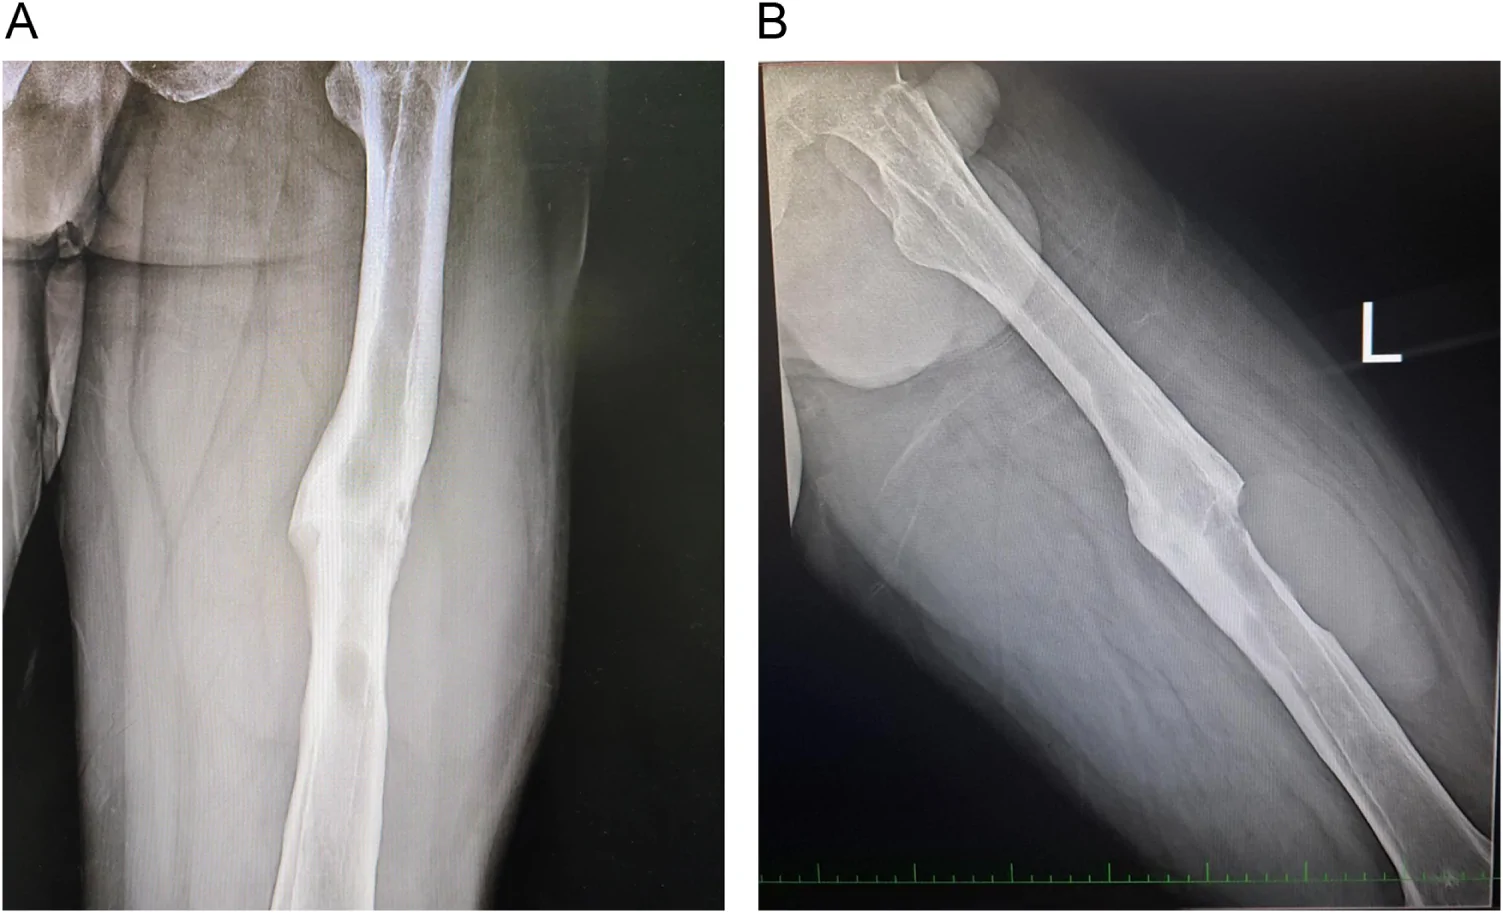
(A and B) Plain radiography of the left femur; anteroposterior view (Fig. 1A) and lateral view (Fig. 1B) show a healed mid-diaphyseal fracture of the femoral neck and osteolysis on the posterior surface.
